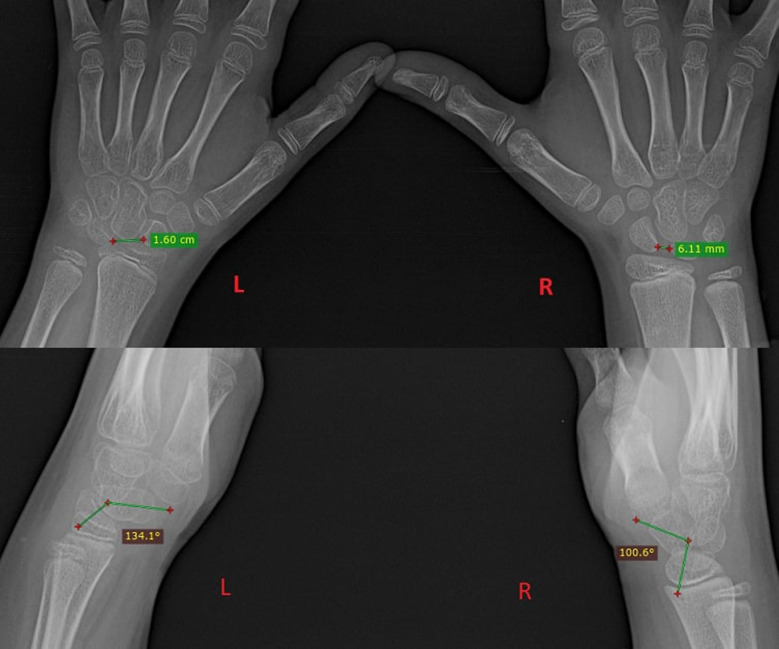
Presurgery X-ray: a comparison between an injured wrist (left) and a healthy wrist (right) in PA and lateral views; measuring the scapho-lunate distance and angle.
